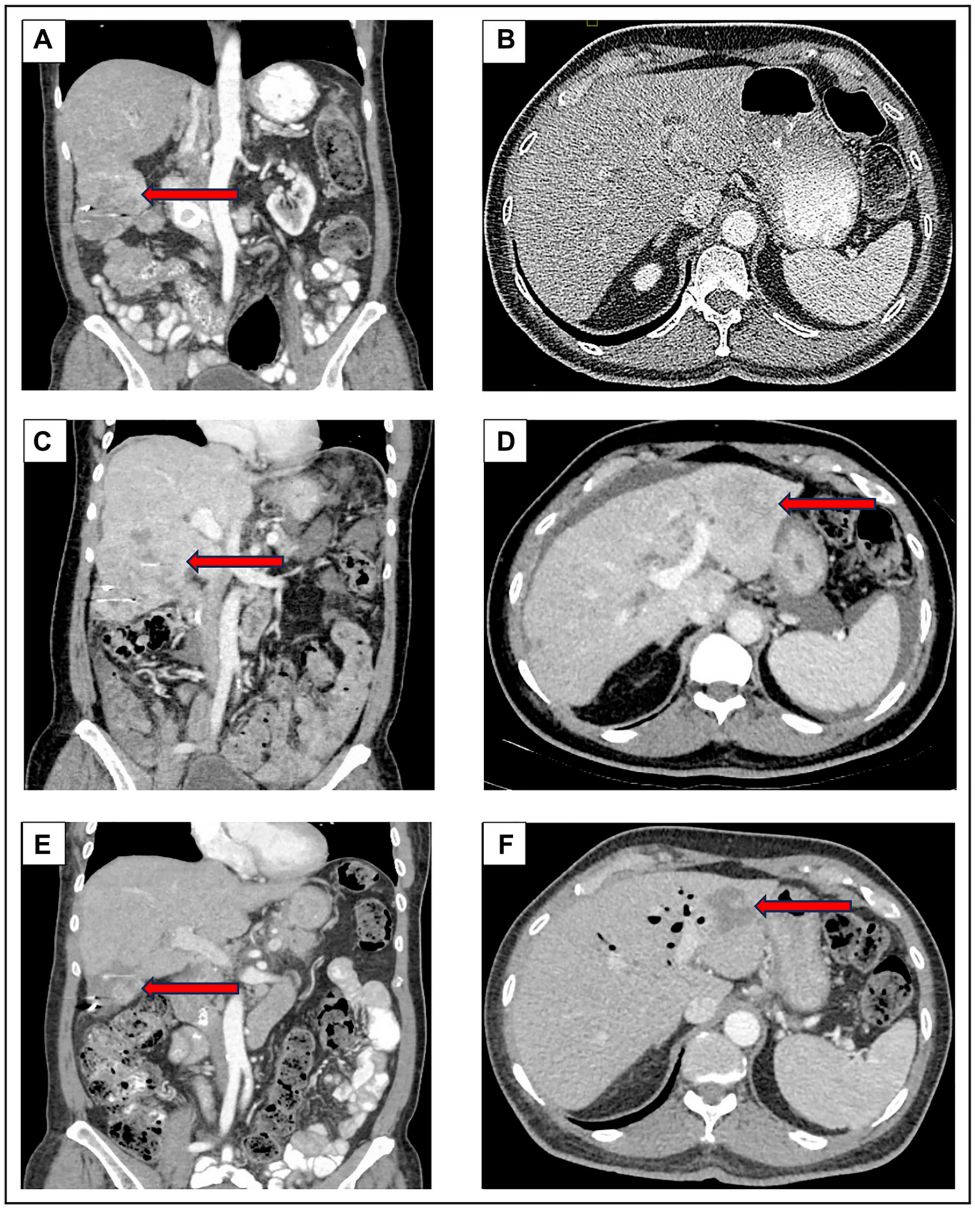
CT Scan on initial presentation to our institution in October 2022 (A, B) demonstrated a right lobe liver lesion measuring 8.2 cm in greatest dimension. In April 2023 (C, D), after six months of treatment with sotorasib, there was an increase in the right lobe liver lesion to 10.2 cm along with a new left lobe lesion measuring 5 cm. In July 2023 (E, F), after four cycles of pembrolizumab, the right lobe liver lesion reduced in size to 5.7 cm and the left liver lobe lesion decreased to 3.5 cm.
